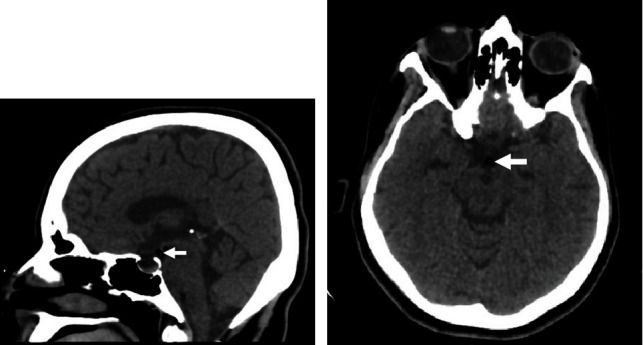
(a) Sagittal brain CT. (b) Axial brain CT. (a) and (b) A hypothalamic lipoma is noted measuring 4 × 4 × 4 mm in maximal dimensions.
